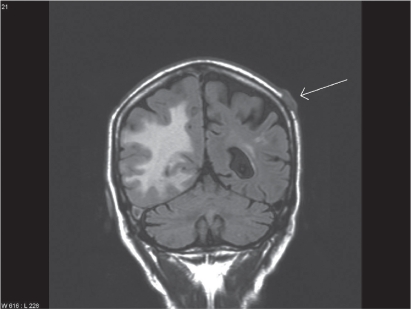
Different section of the MRI examination: cutaneous metastasis indicated by white arrow, note also the edema in the right hemisphere.
